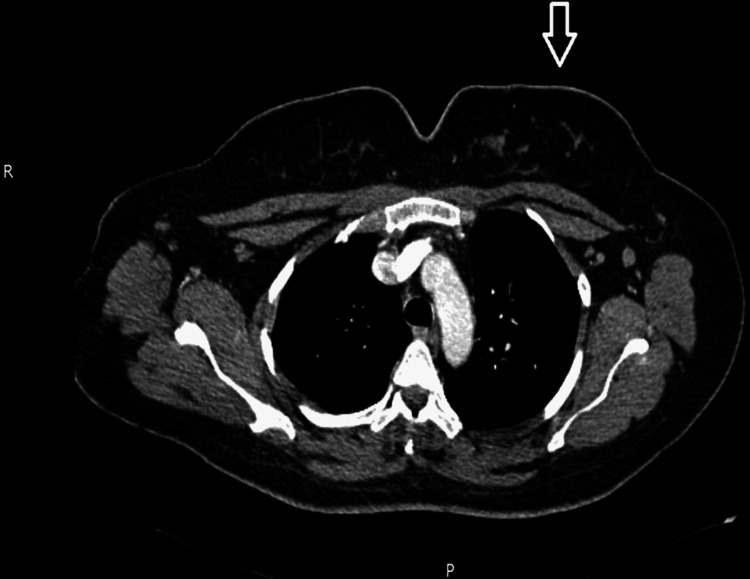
Computed tomography of chest, abdomen and pelvis with contrast was done, which showed the left breast having minimal parenchymal asymmetry
